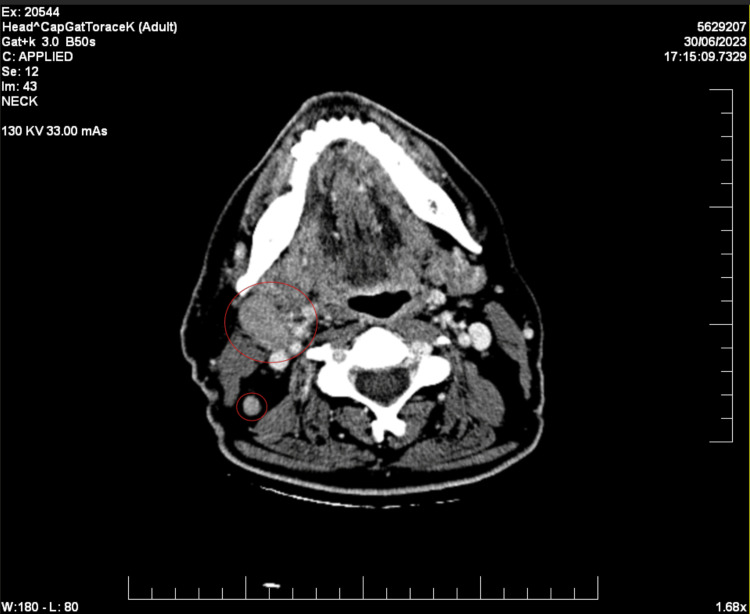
CECT showing lymphadenopathies in the right laterocervical and submandibular regions (red circles). CECT: contrast-enhanced computed tomography
